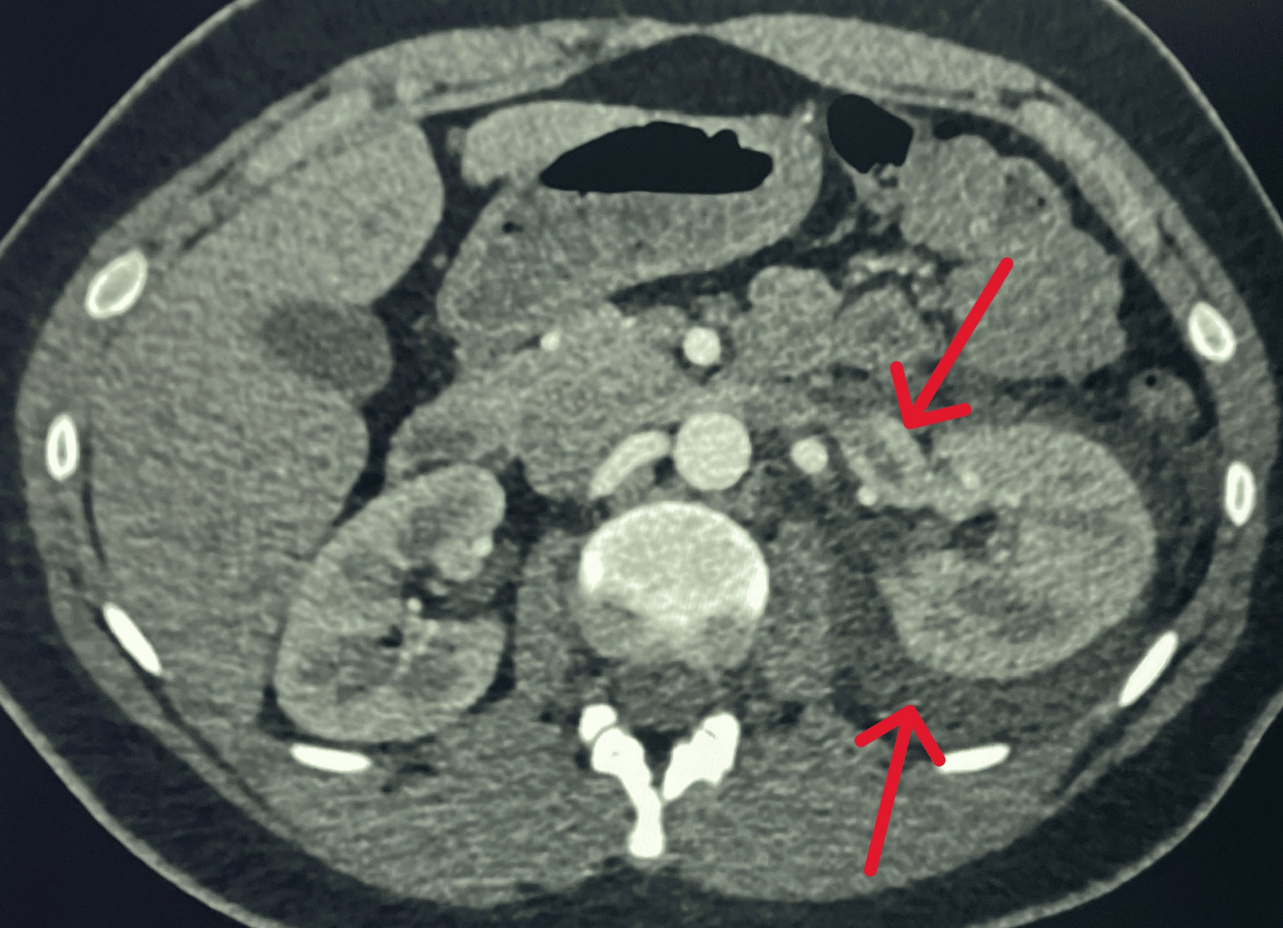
CT abdomen with contrast showing left renal vein engorgement and perinephric stranding The top red arrow indicates left renal vein engorgement, and the bottom red arrow indicates perinephric fat stranding. CT: computed tomography
